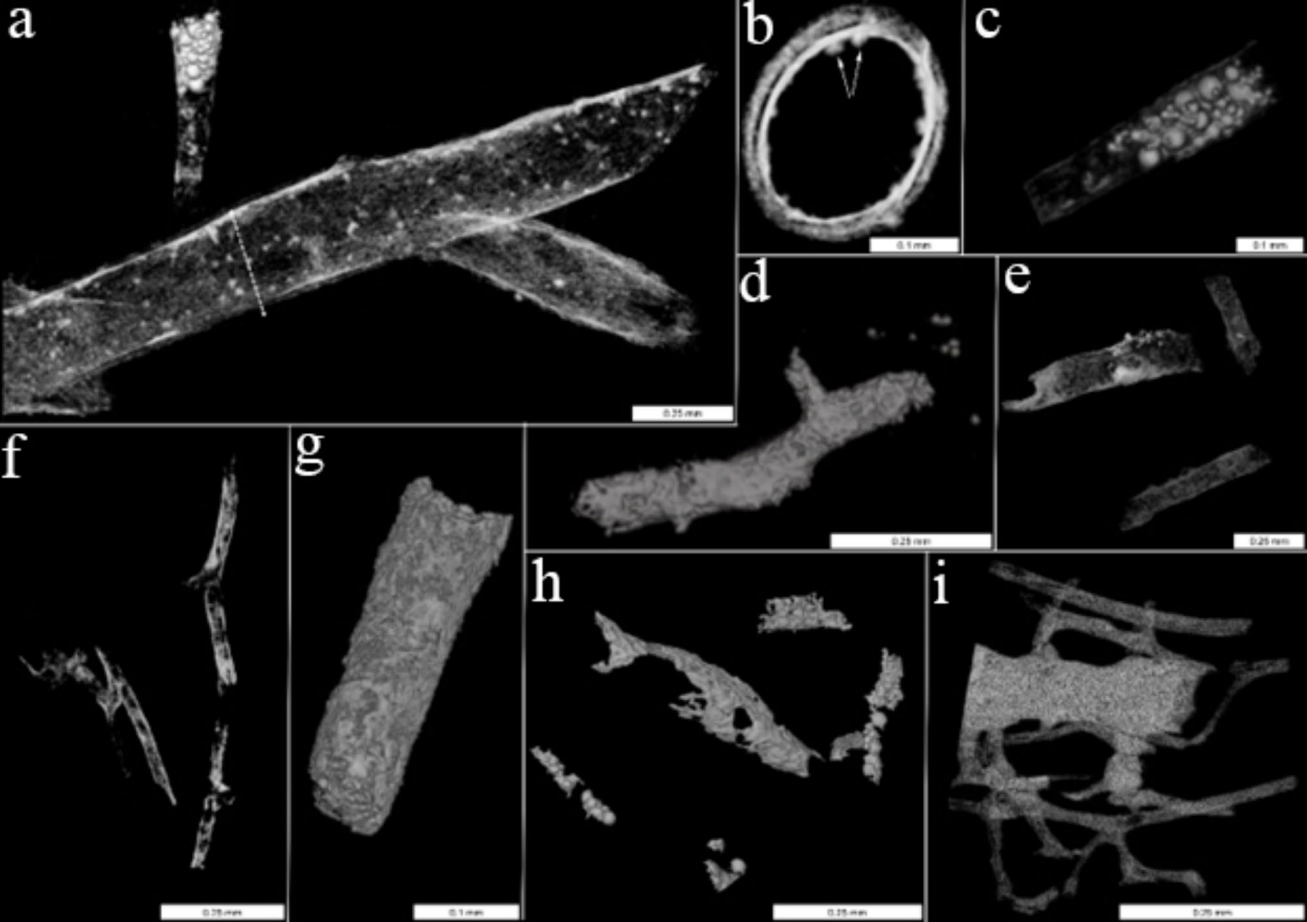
Representative nano-CT images of dinosaur vessels. a–c, MOR 2598. a, overview, shown with a smaller vessel containing abundant rounded spheres. b, Cross-section, taken at the level of dashed line in a. c, vascular inclusions from MOR 2598. d, MOR 10857 showing heterogeneous surface texture and branching of vessels; e, MOR 555/USNM 555000 shows complex vessel walls with varying density; f, MOR 1125 vessels are long, hollow and show branch points for smaller vessels; g, MOR 1126 has dense outer layer, perhaps indicating encrustation; h, MOR 1128 vessels are highly fractured, with vessel on lower left containing dense, spherical vascular inclusions; i Vessels liberated from extant ostrich (*S. camelus*) show greater interconnectivity and more branching of vascular network. Scale bars are as indicated.
